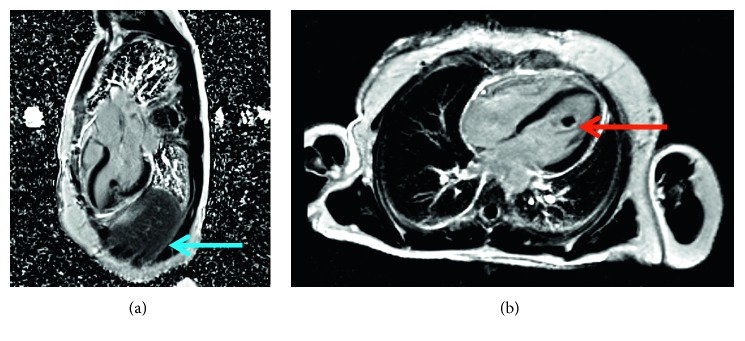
Cardiac MRI demonstrating a new mass infiltrating the left ventricular inferior myocardium, epicardial fat, and pericardium (blue arrow and red arrow).
